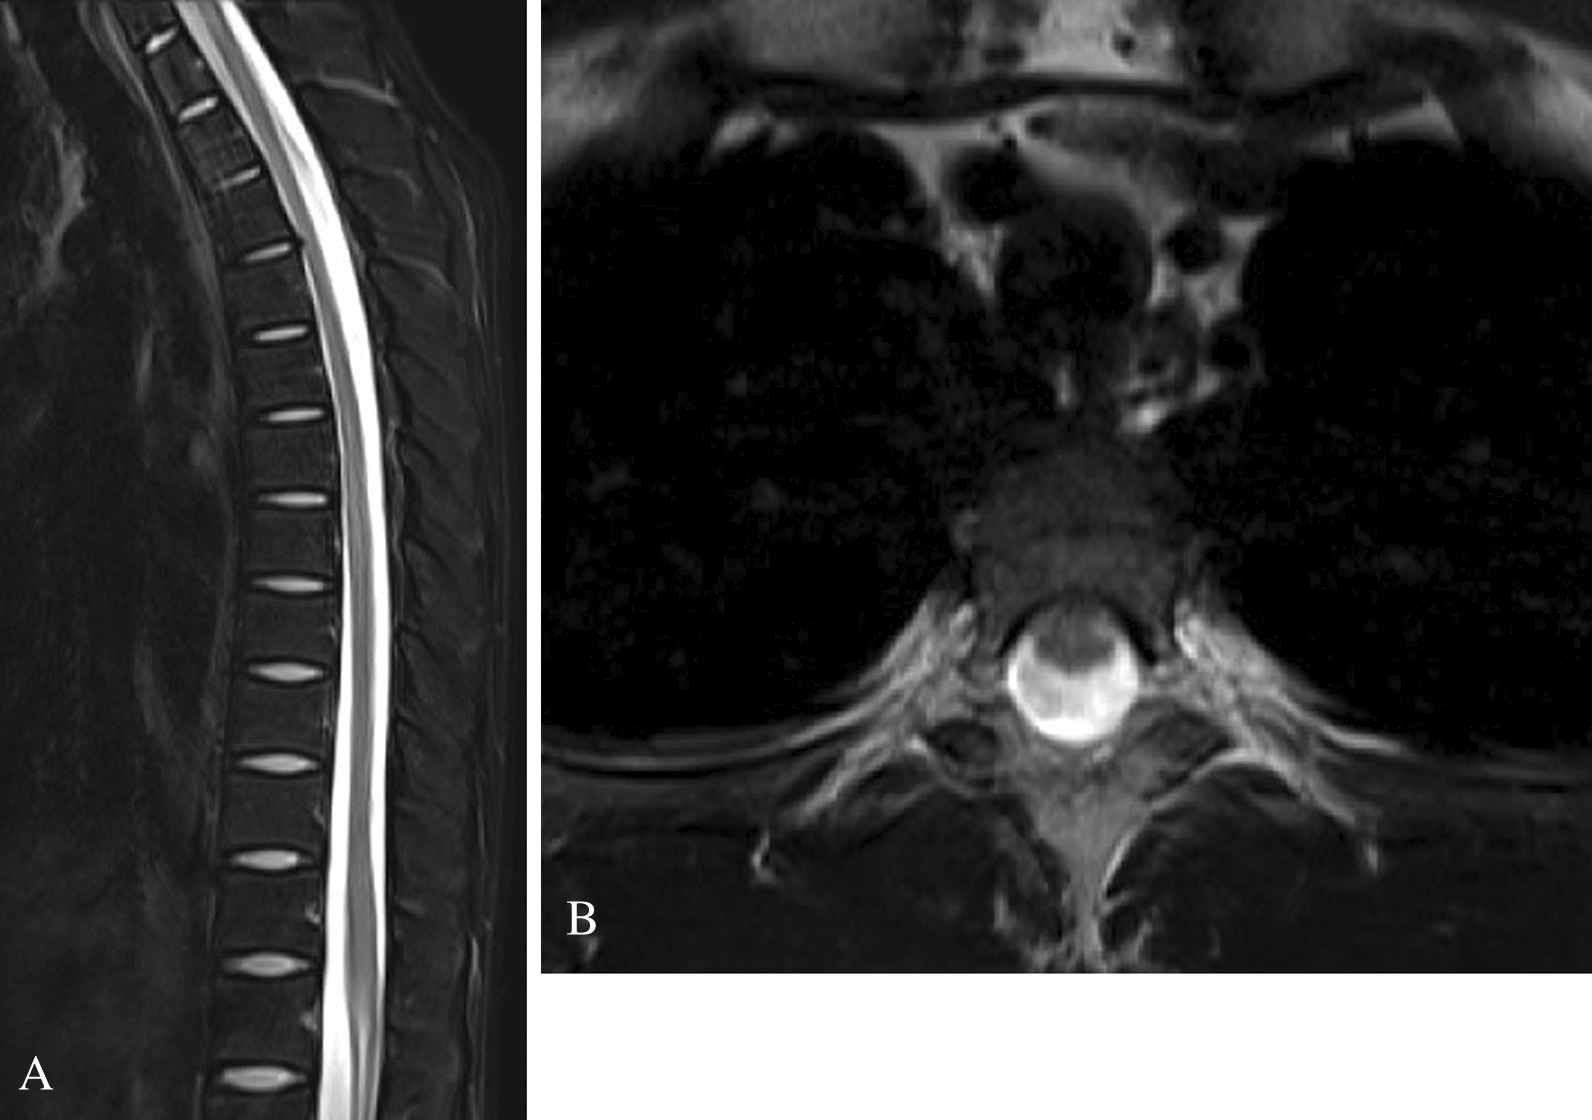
Magnetic resonance imaging (MRI) findings of the cervical and thoracic spine. (a) Sagittal MRI (T2-weighted) of the thoracic spine with tiny herniation of Th4–5 and passage of the spinal cord through the upper and lower vertebrae at the shortest distance. (b) Transverse MRI (T2-weighted) shows decreased spinal fluid in the anterior spinal cord in the Th4–5 region
